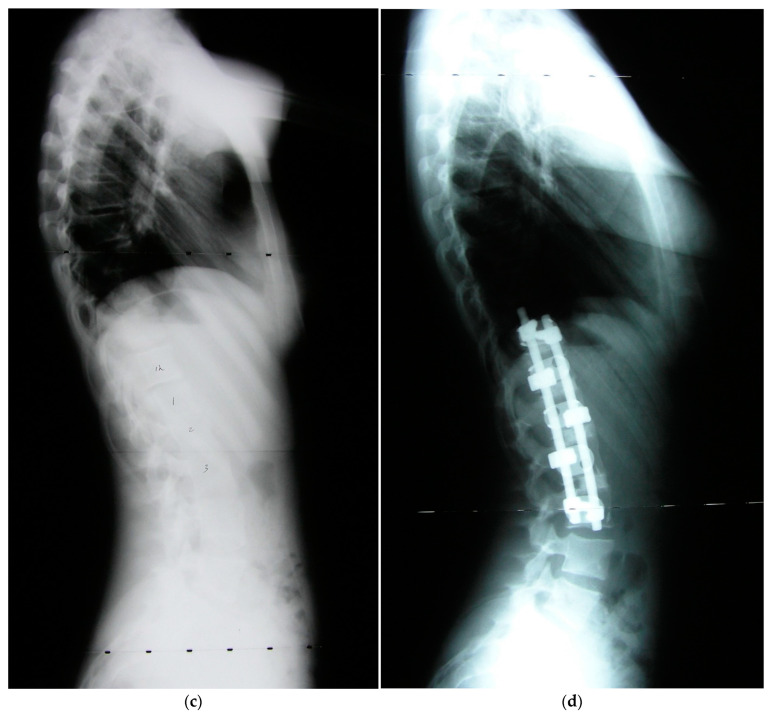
Preoperative coronal and sagittal radiographs (a,c) and postoperative radiographs (b,d). (a) AIS Type 5 preoperative coronal (upright indicated by arrow) radiograph with 60 degrees scoliosis. (b) Postoperative radiograph at one year after traditional convex technique.
